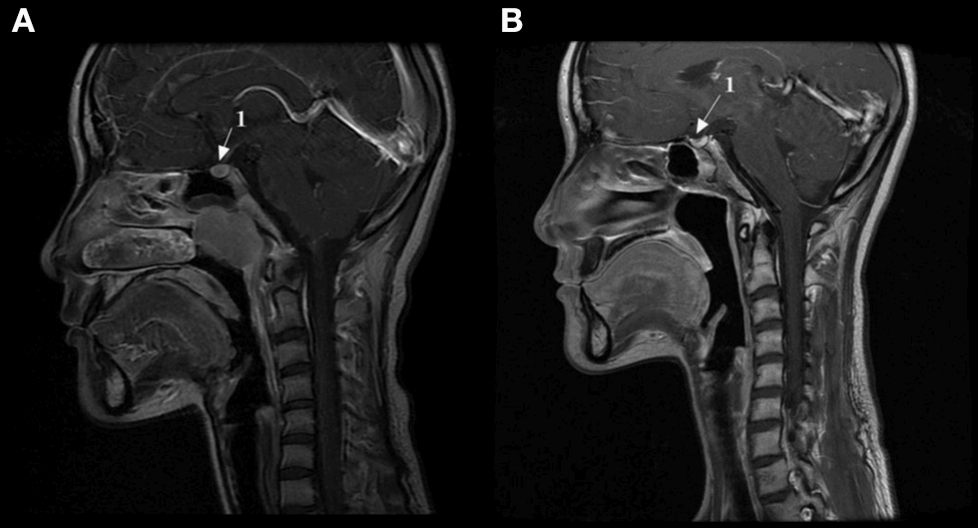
The MRI images of the pituitary gland of one of the childhood NPC patients before and after radiotherapy. (A) MRI image of the pituitary gland before radiotherapy. (B) MRI image of the pituitary gland after radiotherapy. 1, Pituitary gland.
